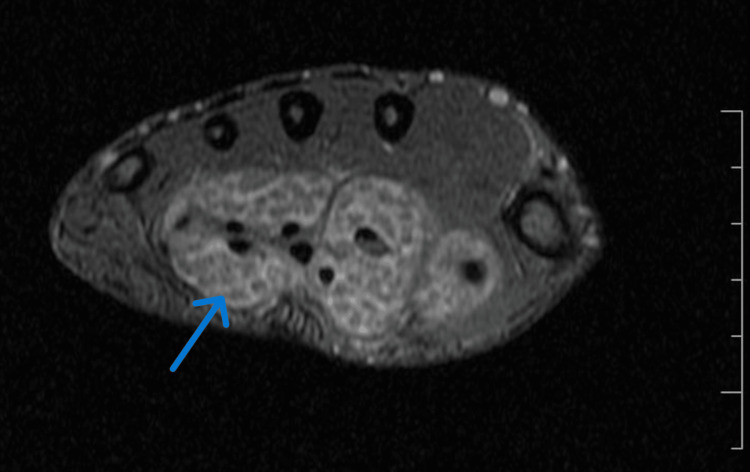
Axial T2* GRE MR image of the hand showing distended radial and ulnar bursae with hyperintense fluid and numerous rice bodies with no blooming artifact GRE: gradient echo
